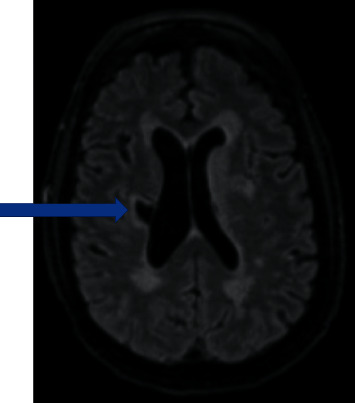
Initial MRI T2 FLAIR delineating chronic right corona radiata and putamen infarcts with ex-vacuo dilation of the right lateral ventricle.
